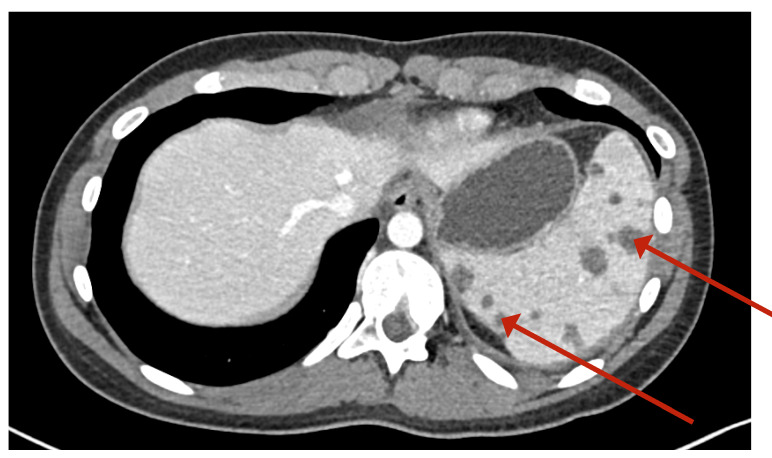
Thoracoabdominal computed tomography. Multiple hypodense nodules of indeterminate appearance are observed in the splenic parenchyma (arrows).
